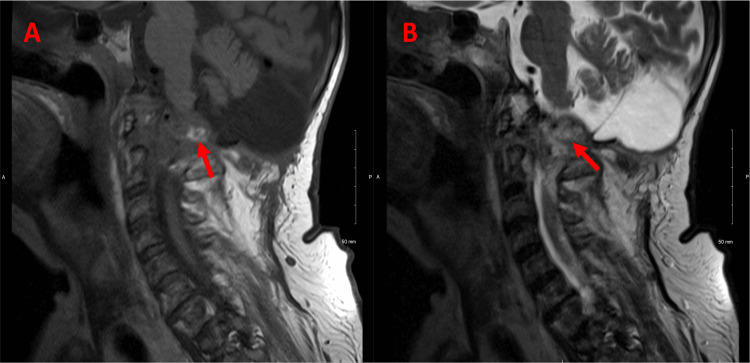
Sagittal magnetic resonance imaging of the patient's head - pre-treatment. (A) T1: Small areas of T1 high-signal thrombus (red arrow) are noted within the aneurysm on the sagittal images. (B) T2: The aneurysm is located at the craniocervical junction and erodes the posterior arch of the atlas and squamous portion of the occipital bone (red arrow).
